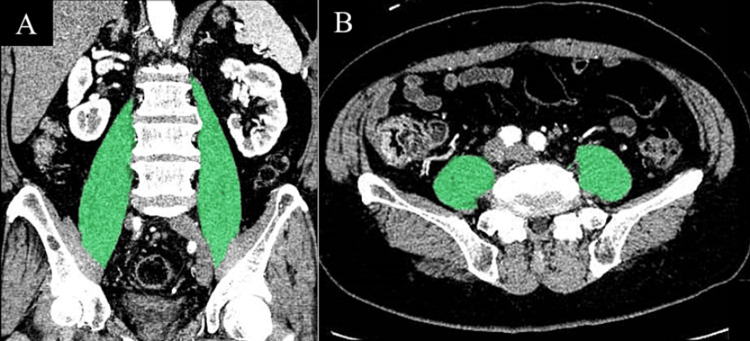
Measurements of the psoas muscle area and volume. (A) The psoas muscle volume was measured at the vertebral level from the diaphragm to the pubic symphysis using automated analysis with Synapse Vincent (Fujifilm, Japan). (B) The psoas muscle area was measured at the middle level of the third lumbar vertebra using a single axial CT scan image.
